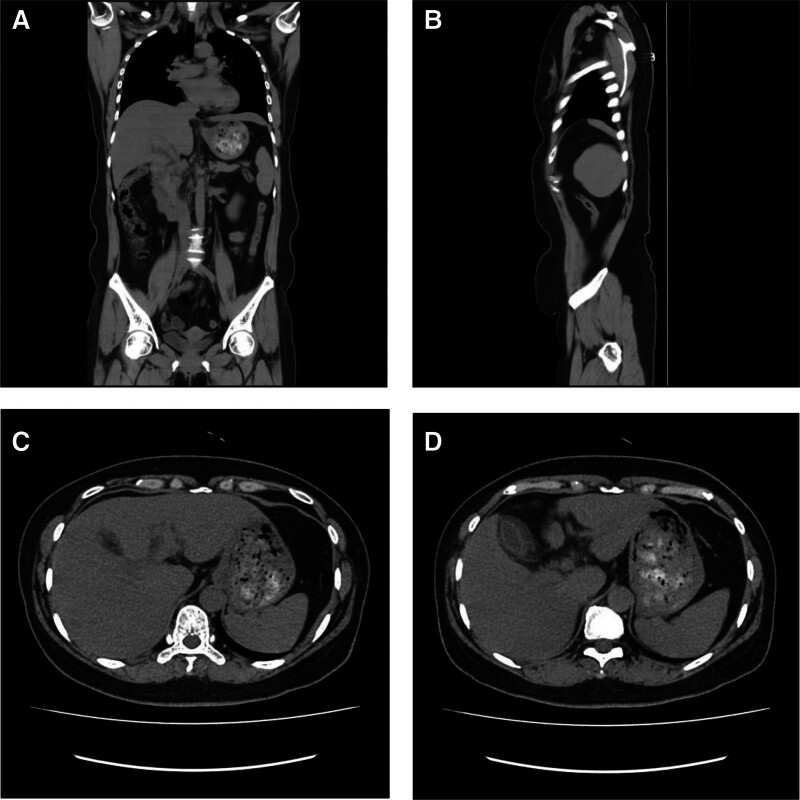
Abdominal plain CT. (a) Coronal. (b) Sagittal. (c, d) Axial. CT = computed tomography.
